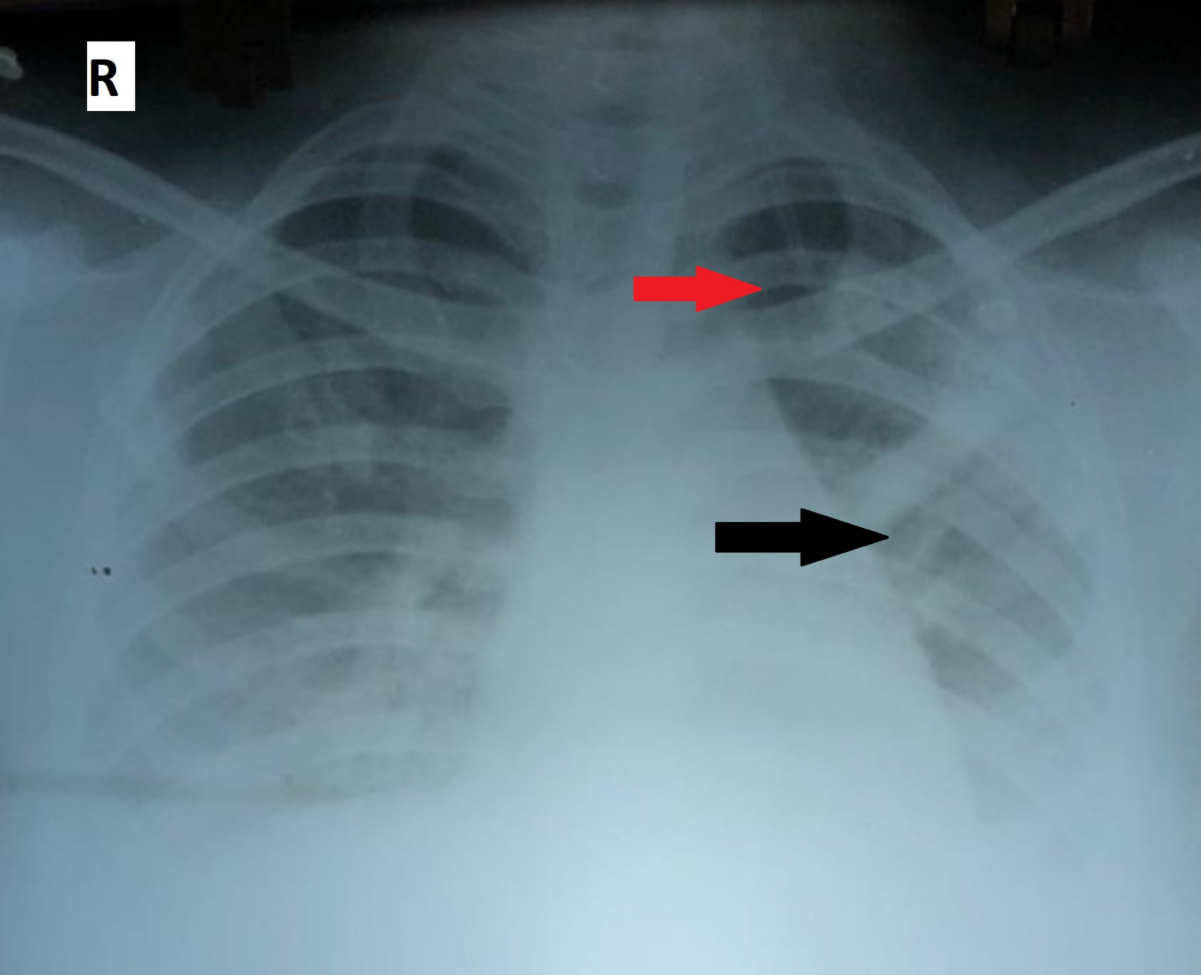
Chest radiograph before discharge showing complete resolution of left upper (red arrow) and mid-zone (black arrow) opacities.
